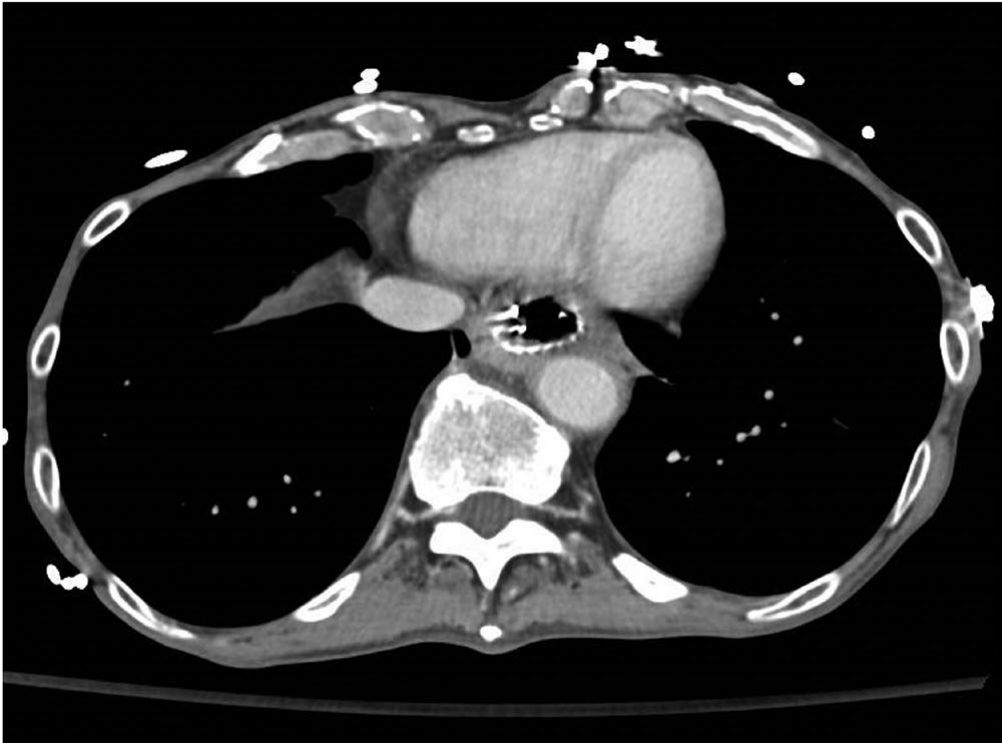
Thoracic and abdominal computed tomography confirming no oesophageal stent migration or cause for the patient’s nausea and vomiting.
